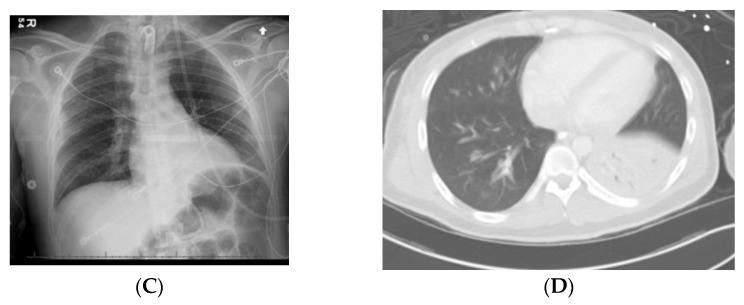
(A–D). (A) CT cervical spine showing C5 burst fracture and area of spinal cord contusion. (B) CT chest showing right pneumothorax with decompression needle in place. (C) Chest X-ray showing dilated loops of bowel from opioid-induced ileus raising the left side of the diaphragm. (D) CT chest showing left lower lobe consolidation and collapse.
